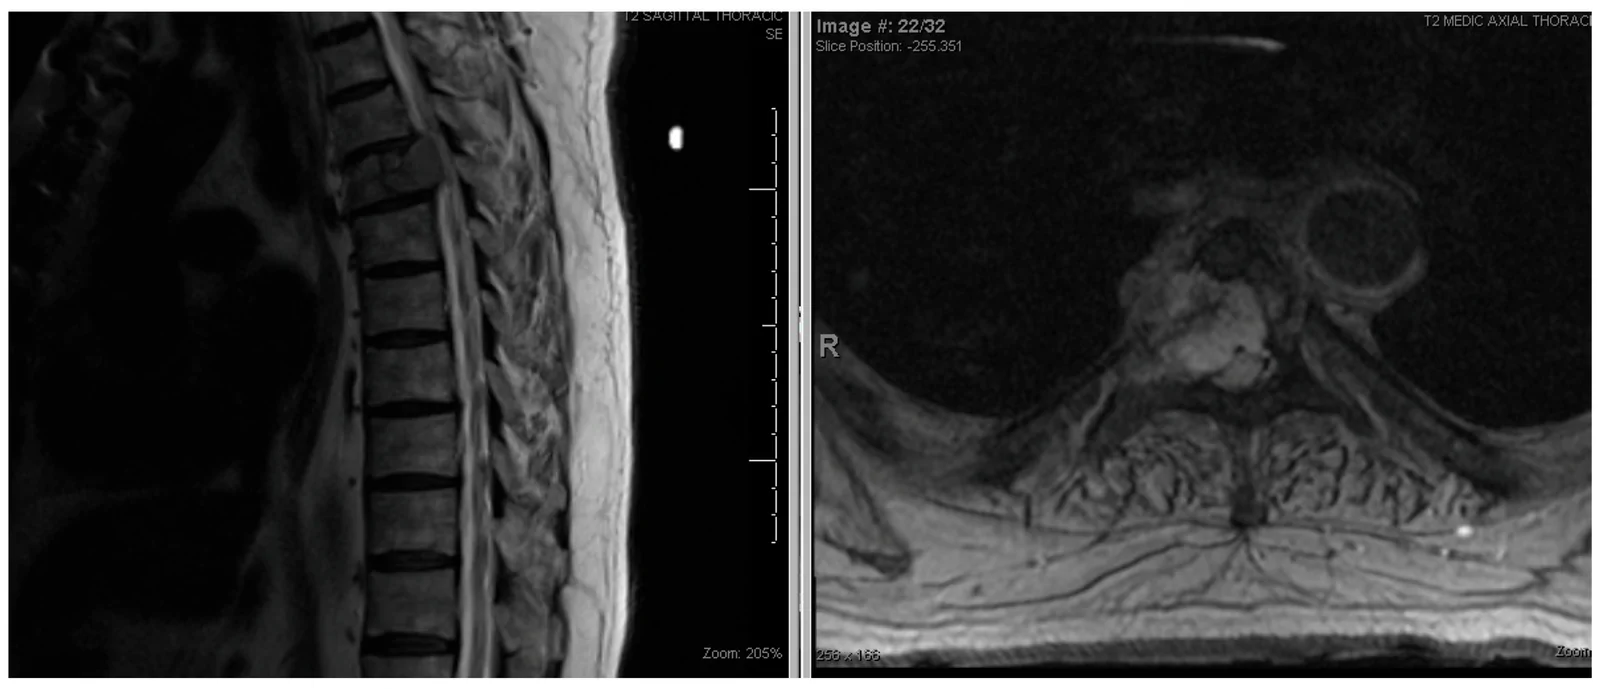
Preoperative T2-weighted MRI (A: sagittal; B: axial) demonstrating extensive T5 vertebral body metastatic lesion secondary to renal cell carcinoma with epidural extension and cord compression in a 76-year-old female.
